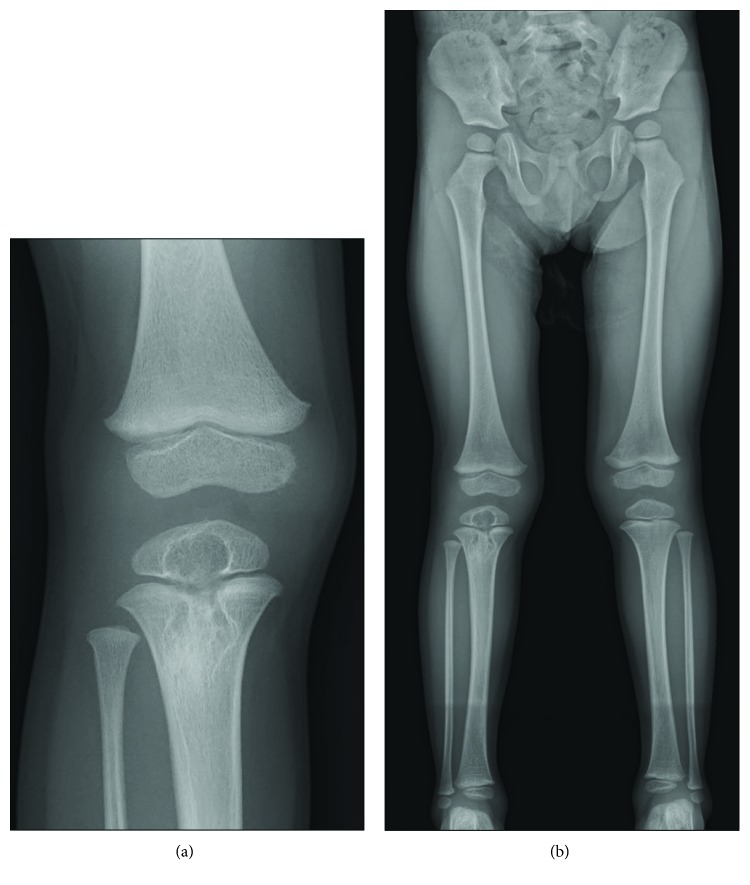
Eight months postoperatively. (a) Plain radiograph showing bone regeneration in the area of curettage and a slight physeal bridge at the right proximal tibia. (b) Growth disturbance and deformation were not seen in a full-length lower-limb radiograph.
